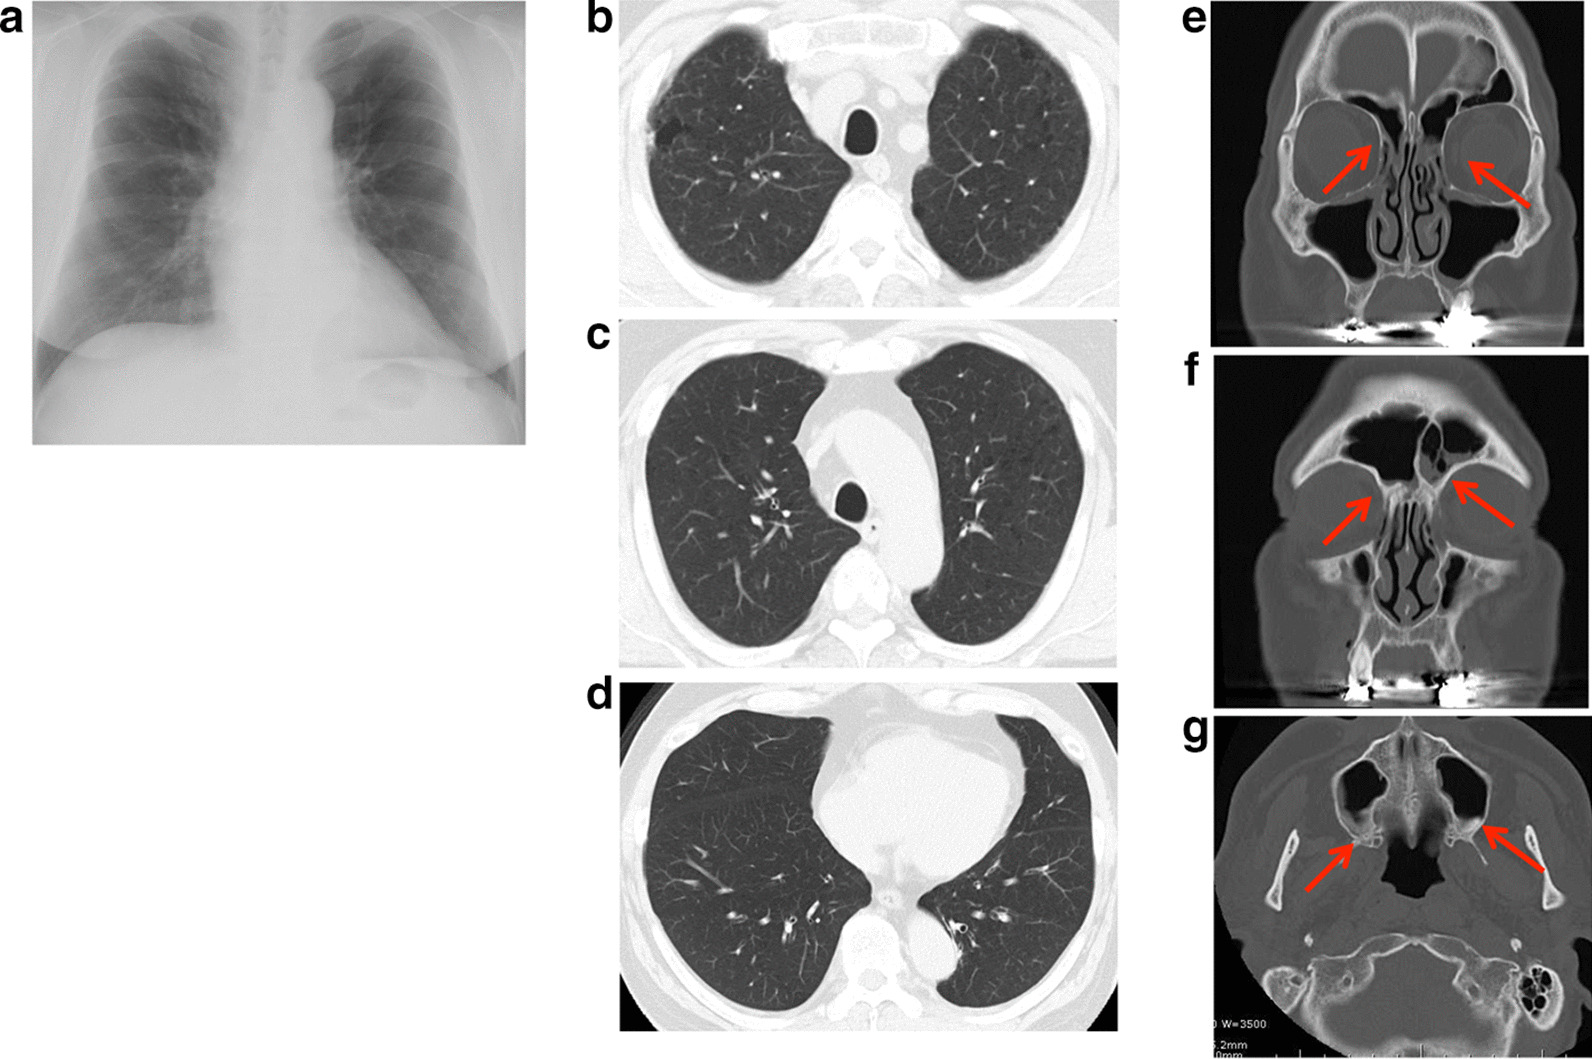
Thoracic radiogram (a), CT scans of chest (b–d) and ethmoid sinus (e), frontal sinus (f) and maxillary sinus (g) after a year from initiation of treatment for EGPA. Chest radiogram (a) shows no any abnormal shadows. Thoracic CT scan of upper (b, c) and lower lobes (d) also does not show any ground-glass opacity. Each arrow in e–g indicates nasal mucosa thickening and fluid collection that was shown in e–g
